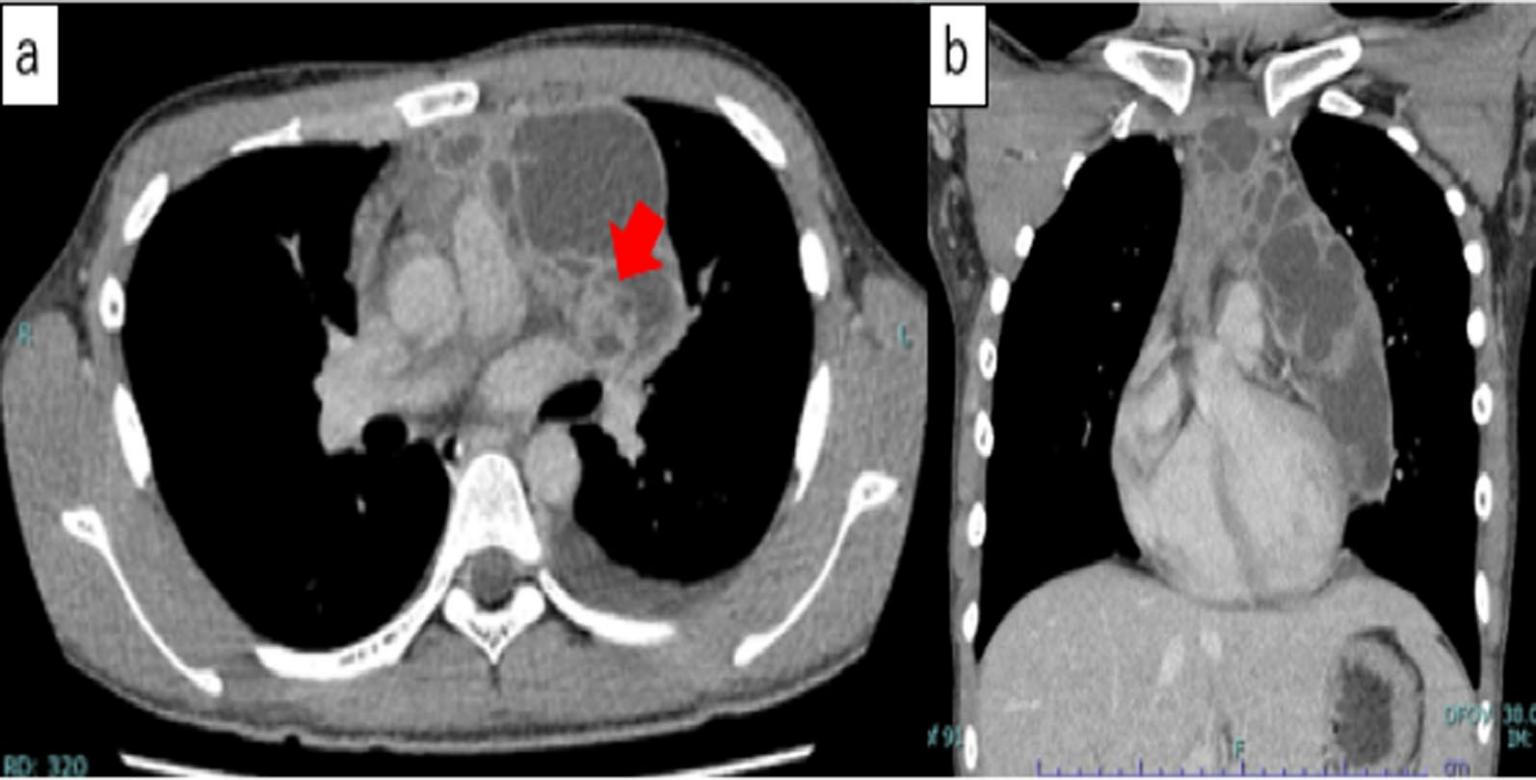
Enhanced CT revealed a multilocular heterogenous giant cyst superior to the anterior mediastinum. Septations with irregular wall thickening and a solid component (red arrow) were observed in the cyst, which had slightly higher CT attenuation than water
